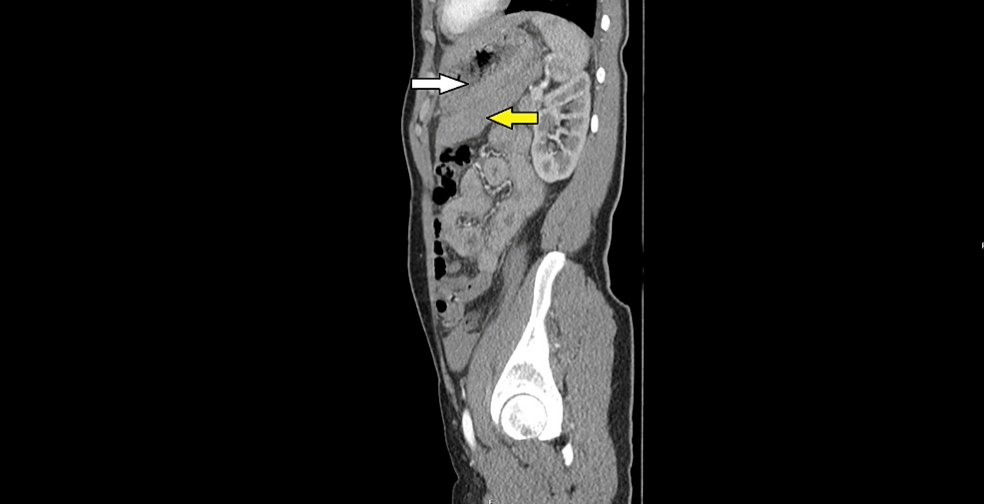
A sagittal CT image of the abdomen and pelvis. The yellow arrow highlights a high-density fluid collection related to the posterior stomach wall, which is denoted by the white arrow
